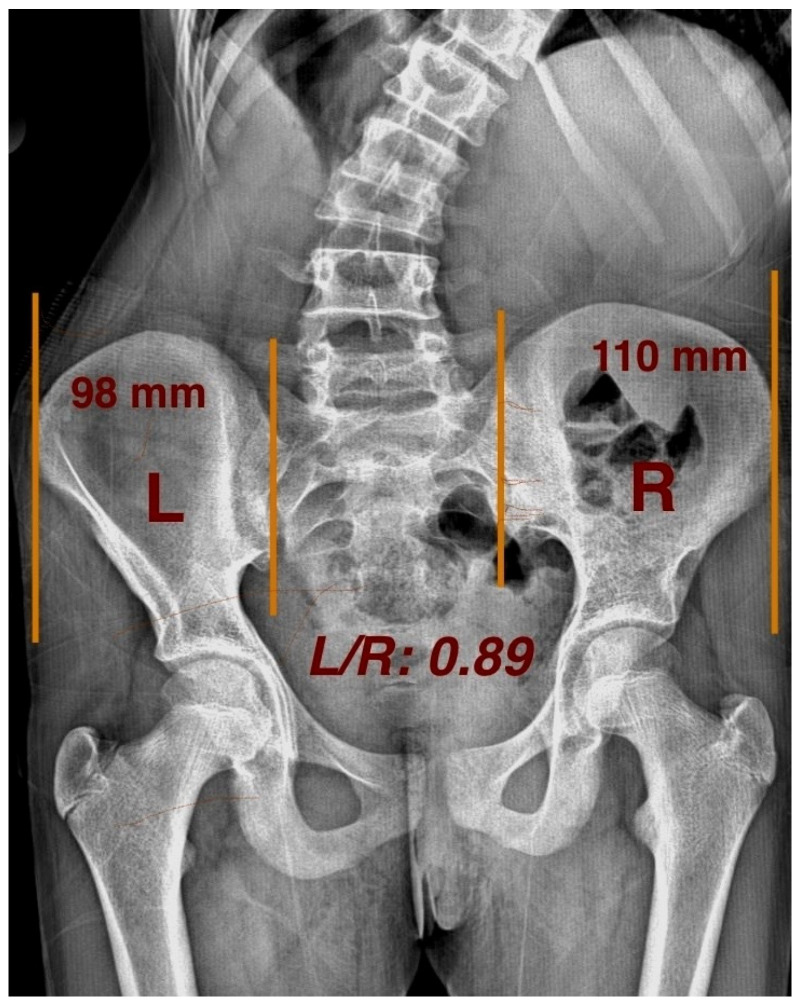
Measuring pelvic axial rotation, and L/R ratio on radiograph.
